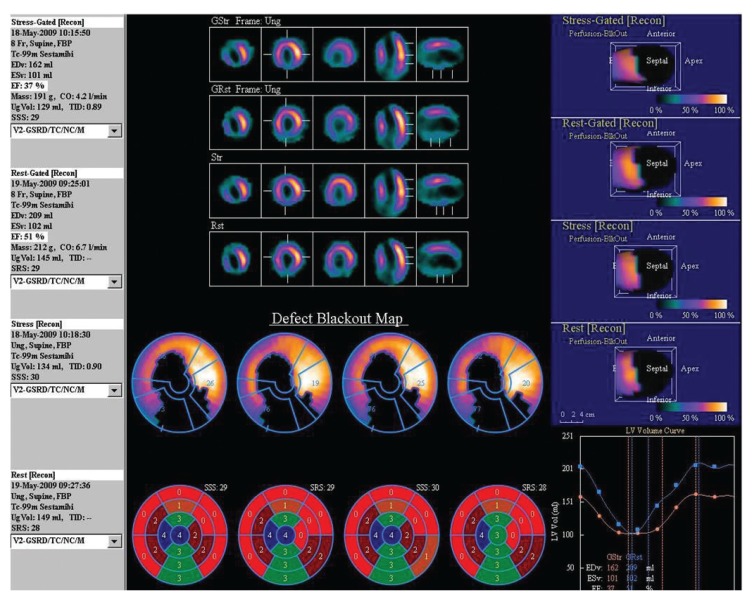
Myocardial perfusion scintigraphy and ‘bullseye’ plots of a 49-year-old male with diabetes, hypertension and significant smoking history. His plasma homocysteine level was elevated (13 μmol/l). ^99m^Tc MIBI imaging at stress and rest showed a significant persistent defect in the anterior wall, apex and inferior wall, with no concurrent ischaemia. The left ventricular ejection fraction was reduced (37%).
